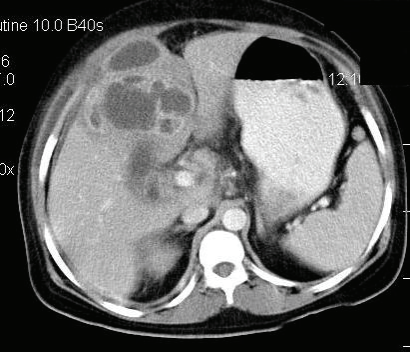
Contrast enhanced computed tomography of the abdomen is showing gall bladder empyema with perforation and formation of liver abscess.
